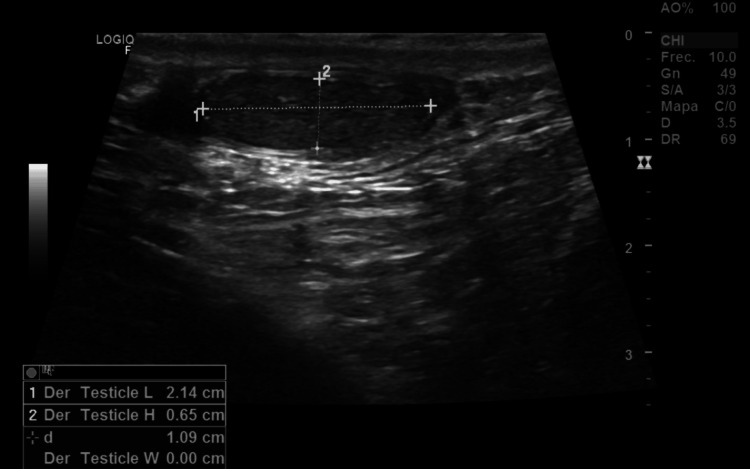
Testicular Doppler ultrasound showing reduced size of the right testicle Ultrasound image demonstrating the right testicle measuring 21 × 6 × 13 mm, indicative of testicular hypotrophy.
